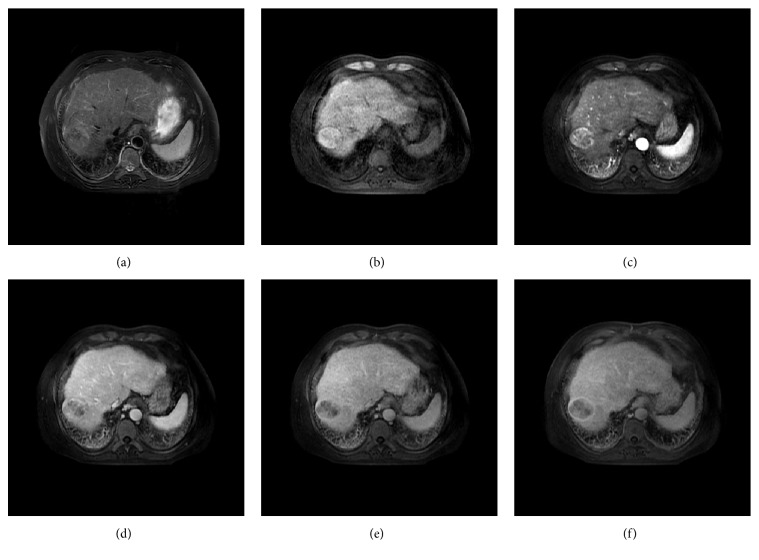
Axial MR images and pathologic image of a 52-year-old man with HCC. (a) A fat-suppressed T2-weighted fast spin-echo image shows an oval-shaped, heterogeneous, slightly hyperintense neoplasm in segment VII with a maximum diameter of 4.6 cm. Axial precontrast (b), late artery phase (c), portal vein phase (d), equilibrium phase (e), and delay phase (f) T1-weighted 3D GRE images show a hyperintense appearance of the lesion on precontrast T1-weighted images (b), obvious enhancement in the late arterial phase (c), and washout in the portal vein phase (d); an enhancing capsule was detectable in the equilibrium phase (e) and was more obvious in the delay phase (f); all of these MRI features are consistent with typical HCC. The tumor was successfully surgically resected and was pathologically confirmed as an MD HCC with no vascular invasion.
